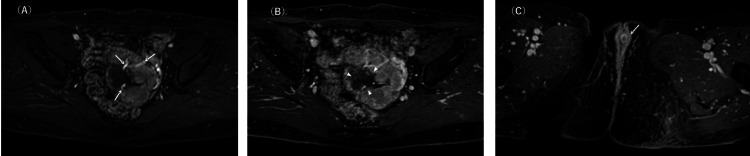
Dynamic contrast-enhanced magnetic resonance imaging of the pelvis On dynamic contrast-enhanced fat-suppressed T1-weighted imaging, the solid parts were intensely enhanced during the early phase (A), and the enhancement persisted in the delayed phase (B). The vasculature in the mass revealed prominent enhancement (A, arrows), corresponding to flow voids on non-contrast magnetic resonance imaging. The central part showed no enhancement and was more clearly visualised in the delayed phase (B, asterisk), indicating cavitation. The areas between these two parts were weakly enhanced (B, arrowheads), suggesting oedematous tissue. An enlarged clitoris was also noted (C, arrow).
